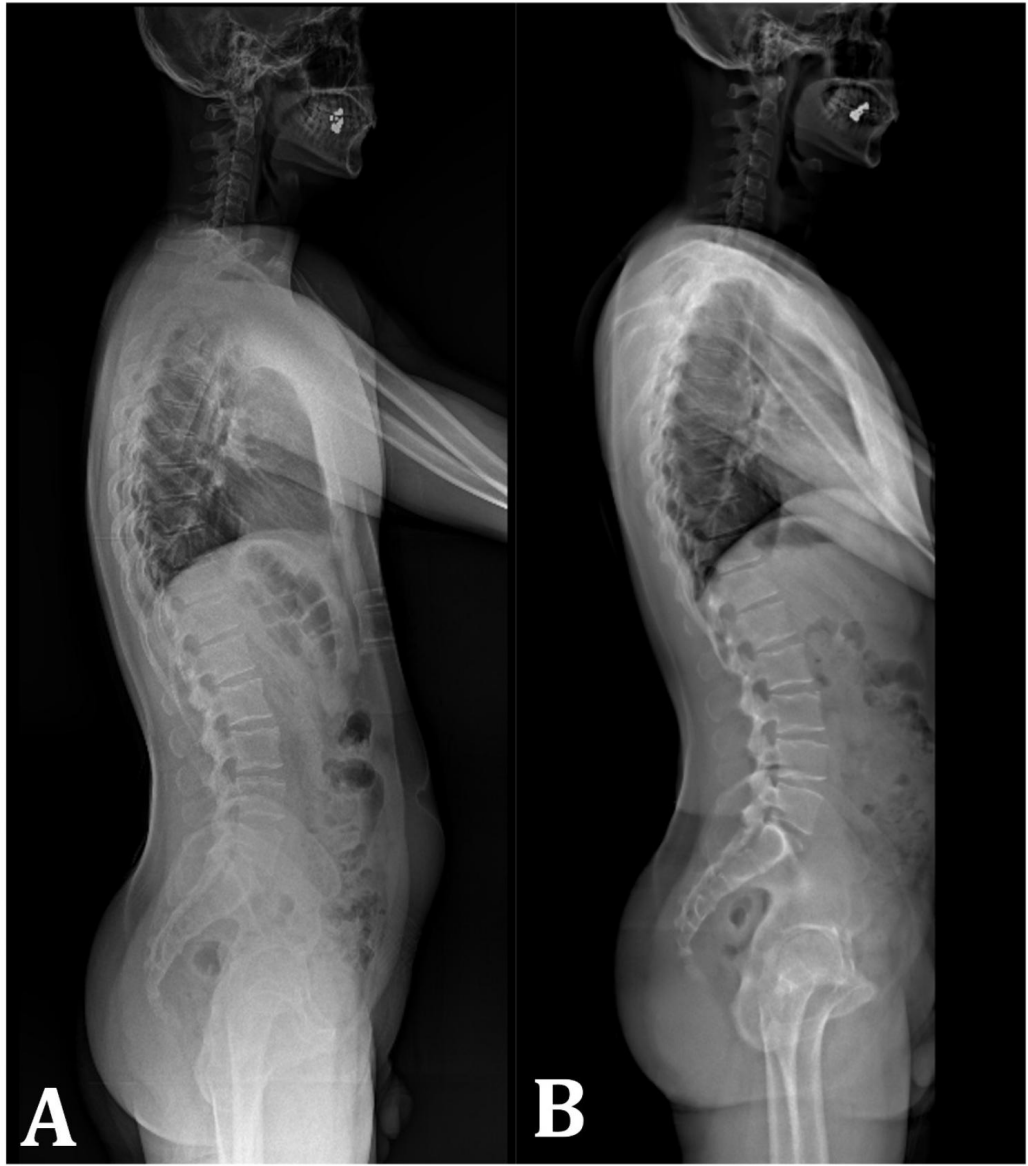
(a) Conventional C1S1 radiograph. (b) EOS^®^ image
